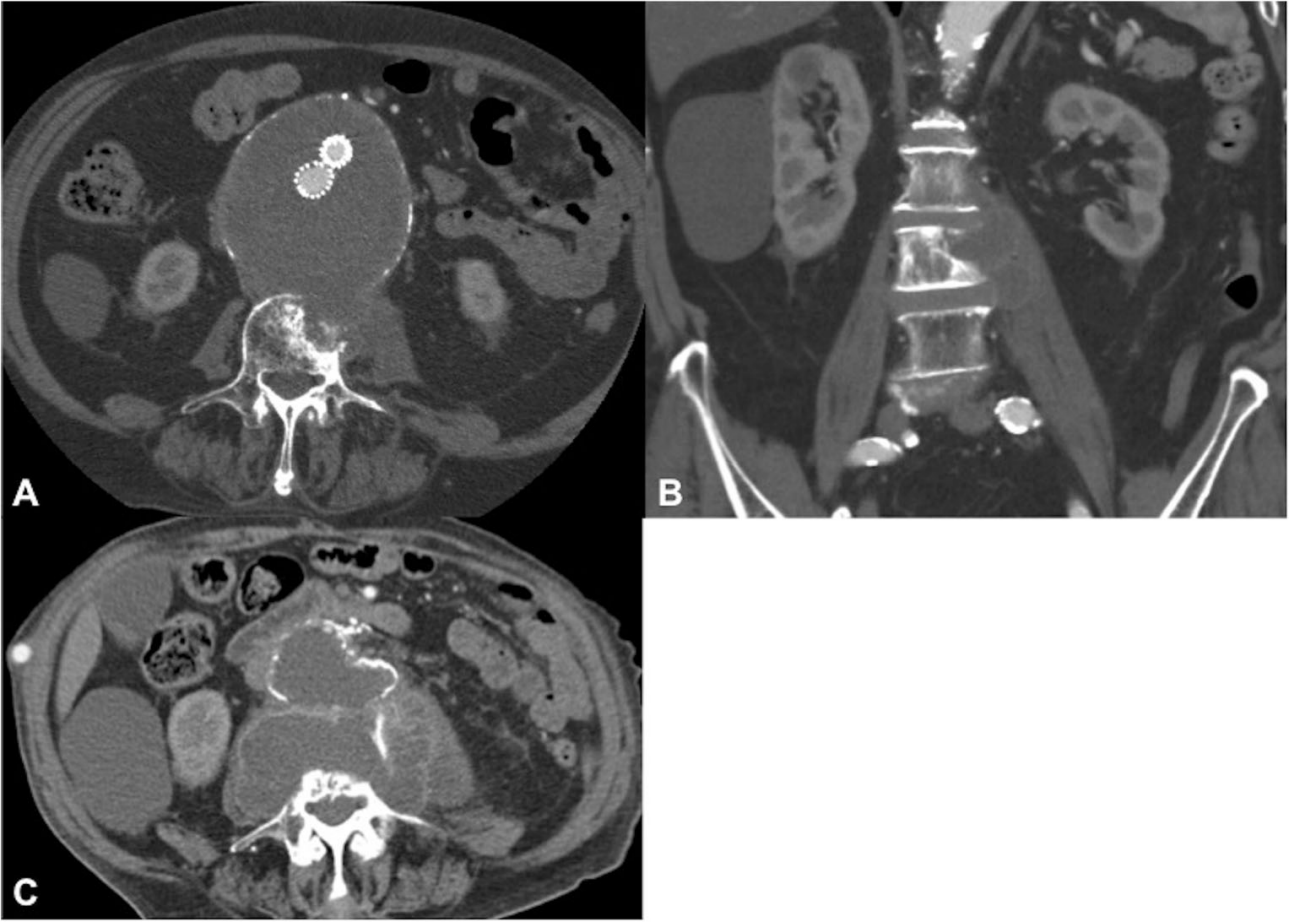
An 87-year-old man (patient 1). a Axial and b coronal CT 19 months following endovascular aortic aneurysm repair (EVAR), demonstrating L3 anterolateral vertebral body and superior endplate erosion. Peripherally enhancing paravertebral/left psoas abscess is contiguous with the markedly expanded native aneurysm encasing the stent graft. c Axial CT after a further 12 months with interval stent graft removal and axillobifemoral bypass. There is now more progressive vertebral body destruction with a contiguous abscess now involving the native excluded aneurysm sac, paravertebral soft tissues and replacing both psoas muscles
